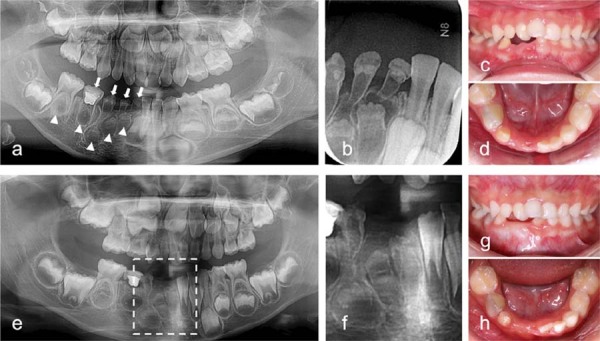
Radiographic and clinical presentation of a 4-year-old girl with ROD in the mandibular right quadrant. (a, b) Initial assessment: Panoramic (a) and periapical (b) radiographs taken on the first visit. White arrows highlight the affected primary teeth, whereas arrowheads indicate potentially involved permanent teeth. (c, d) Post-extraction intraoral views: Clinical images showing the mandibular right quadrant following the extraction of teeth 81 and 82 due to severe mobility. (e, f) Two-year follow-up imaging: Panoramic radiograph (e) and a magnified localized view (f) showing the developmental progress and radiographic changes in the region. (g, h) Two-year follow-up intraoral views: Clinical images indicating the soft tissue and dental status of the affected region at the two-year mark.
